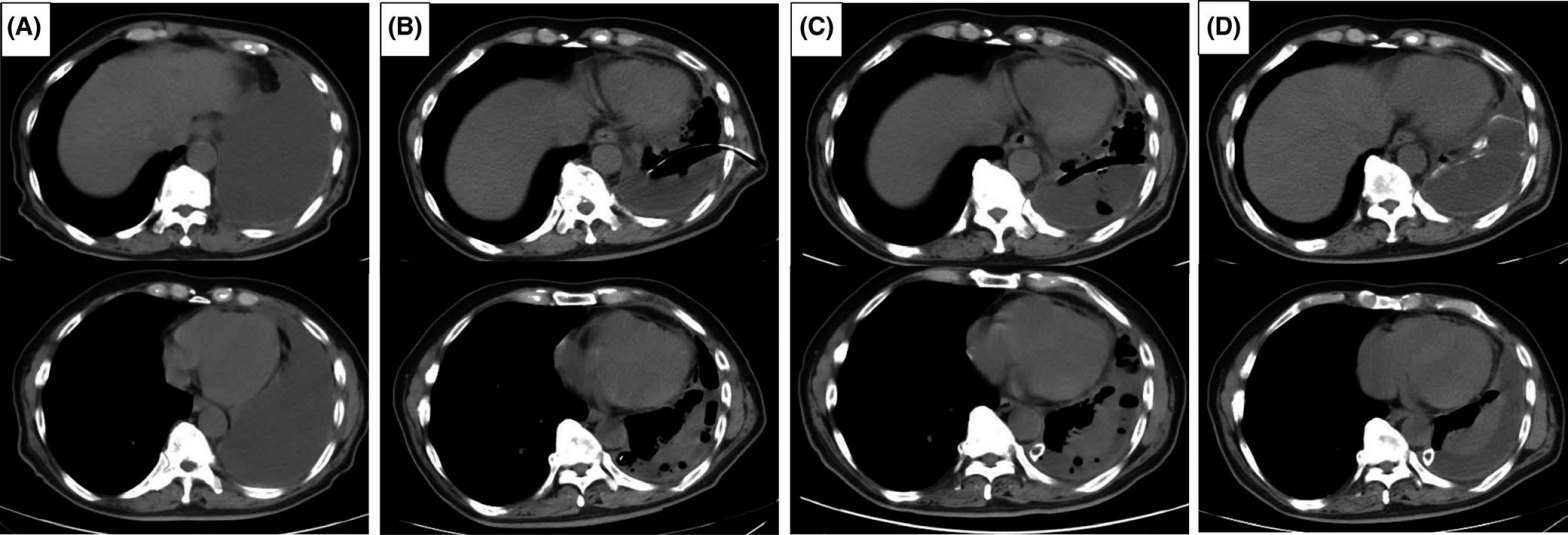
(A) Computed tomography (CT) performed before the drainage showing left pleural effusion. (B) CT after the drainage showing insertion of an actual chest tube. (C) CT performed 1 wk after talc pleurodesis showing the “ghost” chest tube. (D) CT 2 mo after pleurodesis showing the remaining “ghost” chest tube
